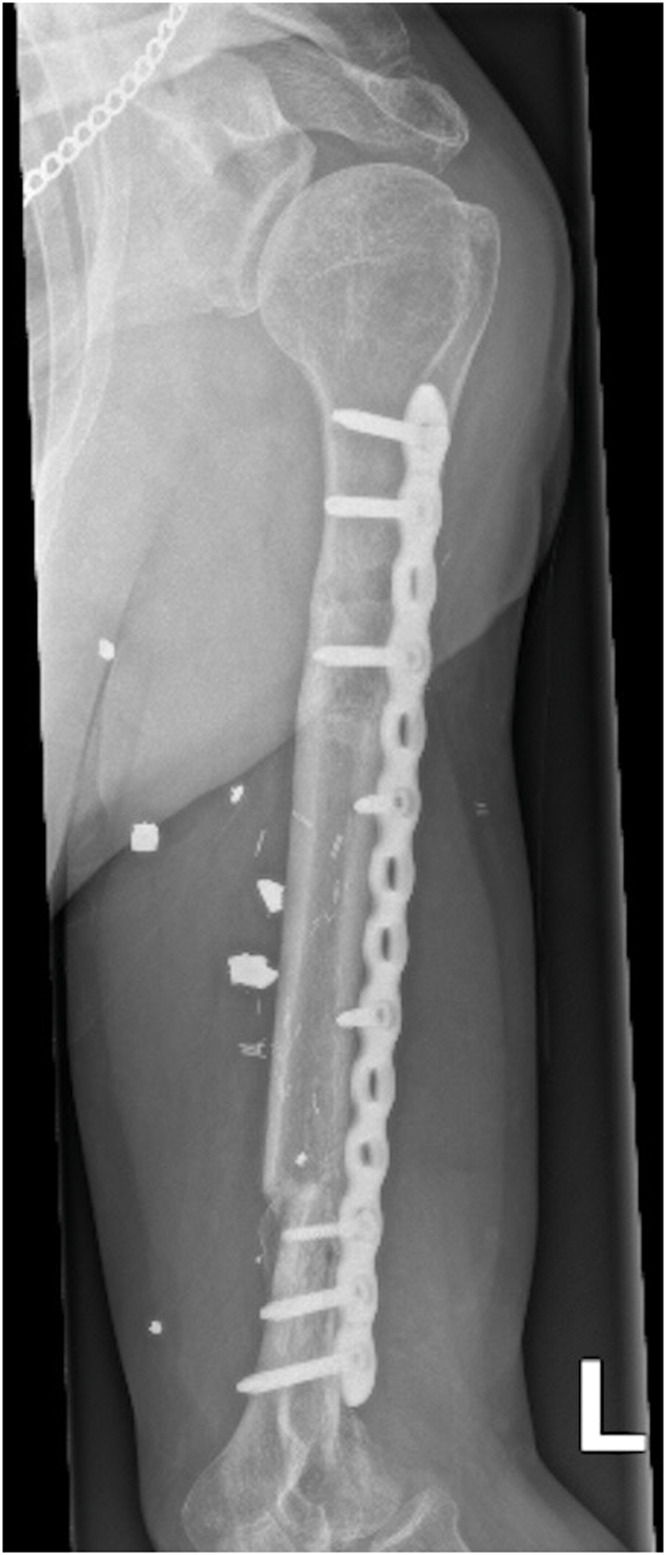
Eleven-month follow-up imaging. Single-view radiograph of the left humerus demonstrating complete consolidation of the vascularized tibial graft with maintained alignment and stable plate osteosynthesis.
